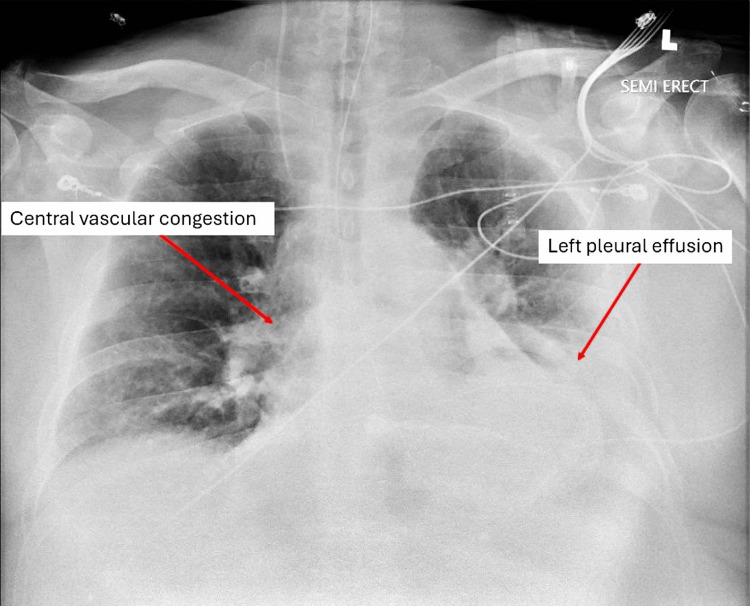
CXR from July 9 showing central vascular congestion and a left pleural effusion. CXE, chest X-ray
